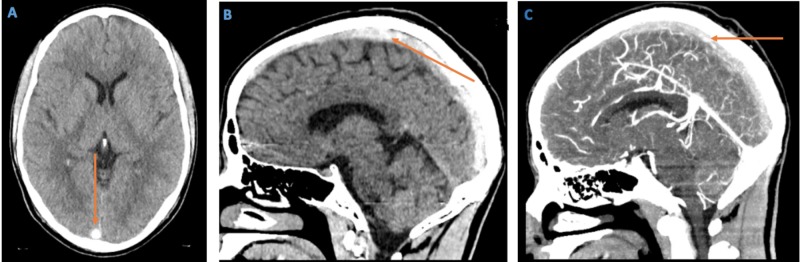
Computed tomography (CT) of the head without contrast (axial and sagittal views) and CT angiogram of the head A) CT head without contrast (axial view) showing hyperdensity at the torcula; B) CT head without contrast (sagittal view) showing hyperdensity at the superior sagittal sinus; C) CT angiogram (brain window) demonstrates extensive hyperdensity in the superior sagittal sinus.
